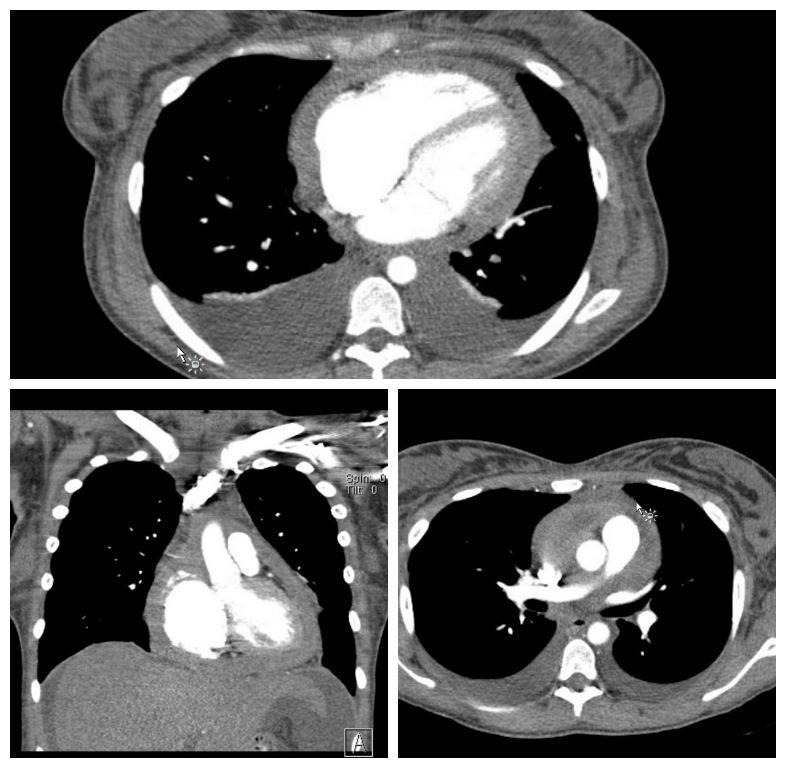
The different views and sections of a computed tomography scan showing a markedly thickened pericardium and level 1 thoracic adenopathy.
